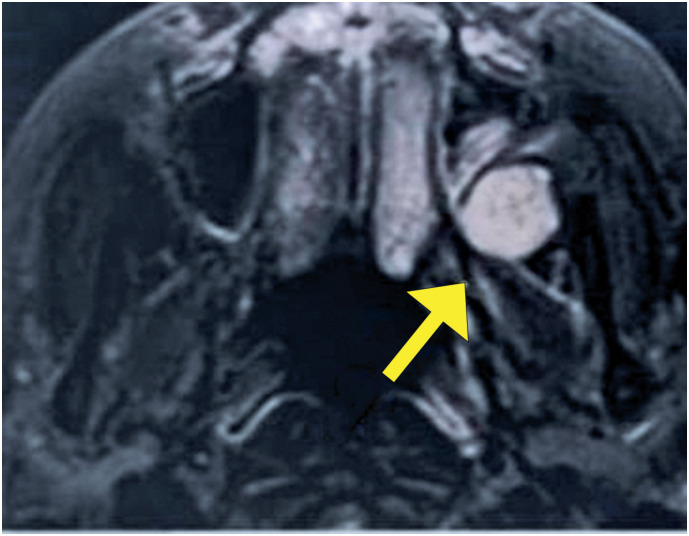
Skull magnetic resonance imaging in an axial section reveals a nodular, solid lesion in the masticatory space on the left, posterior to the ipsilateral maxillary sinus, with a volume of 2.0 × 1.8cm and hyperintensity on T2/ fluid-attenuated inversion recovery
